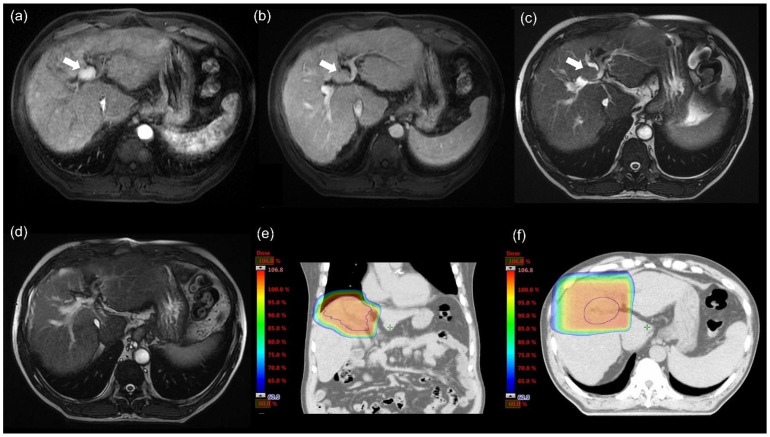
Tumor response of hepatocellular carcinoma. The tumor thrombus (white arrow) in bile duct showed washed-in in the arterial phase (a) and washed-out in the venous phase (b) of magnetic resonance imaging (MRI). (c) Tumor thrombus (white arrow) in bile duct could also be identified in T2 phase of MRI. (d) Irradiated tumor thrombus had a complete response one month after proton beam therapy (PBT). (e) The coronal view of the treatment plan. (f) The axial view of the treatment plan.
